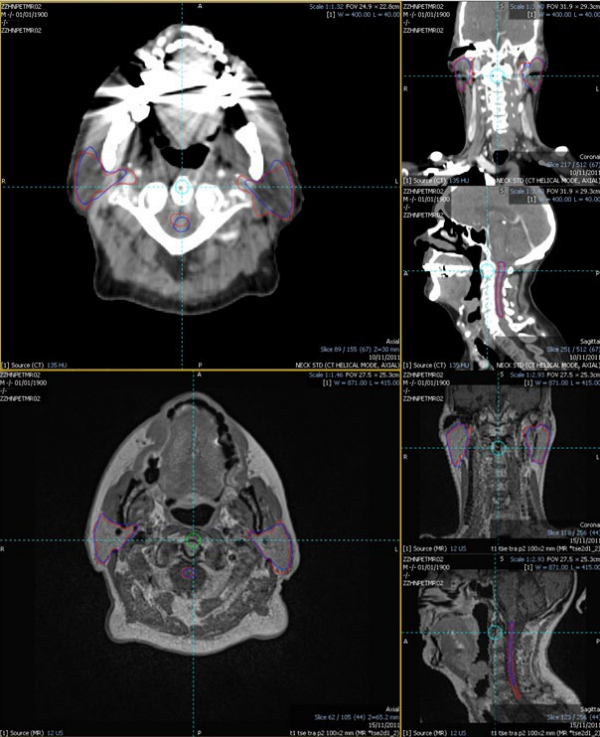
Example manual contours (red) and autocontours (blue) for the spinal cord as well as left and right parotids for Patient ‣2. Top images are CT showing large dental artifacts and poor autocontours, and bottom images are MRI showing more accurate autocontours.
